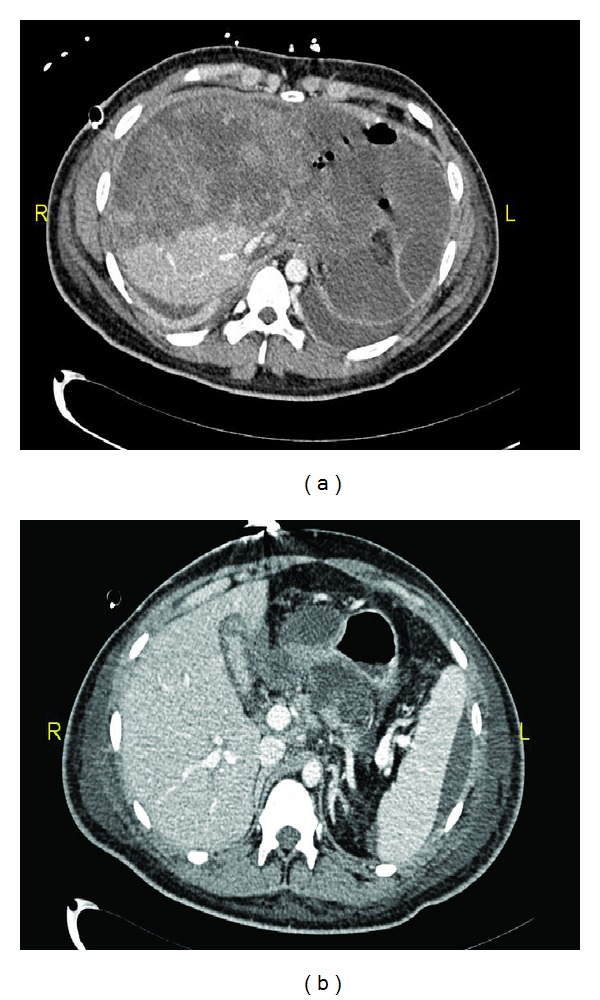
(a) Admission IV contrasts abdomen CT scan showing extensive liver injury with active blush; (b) fluid around spleen and liver.
